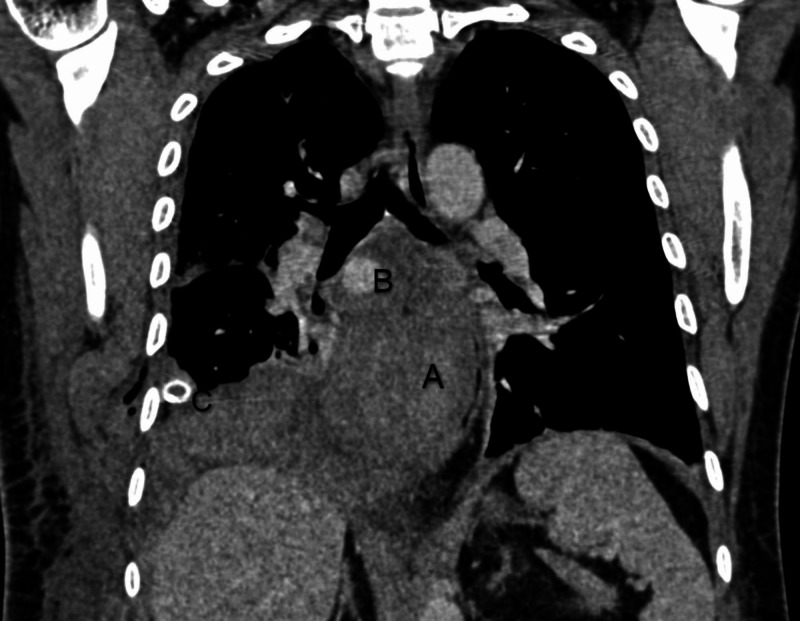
Coronal computed tomography (CT) section showing mediastinal hematoma with contrast-filled pseudoaneurysm adjacent to bronchus intermedius and chest tube in right pleural space. A: mediastinal hematoma B: contrast-filled pseudoaneurysm C: chest tube in right pleural space
